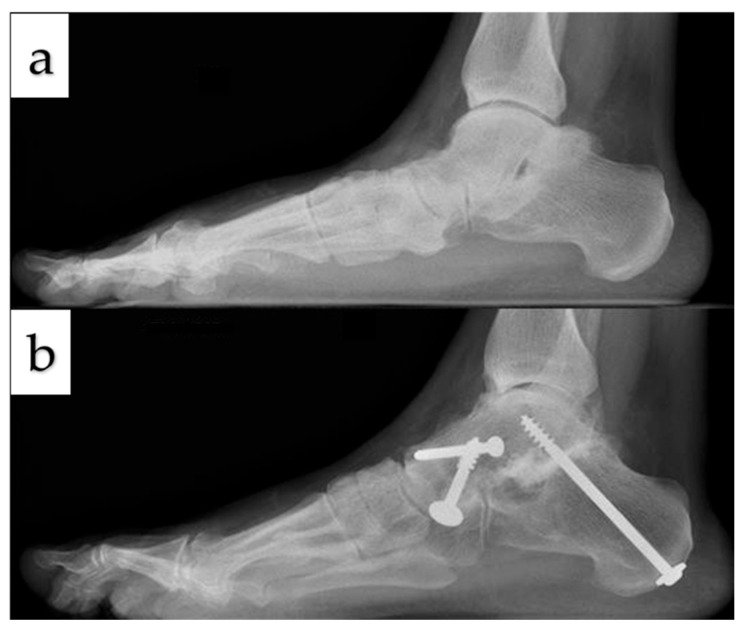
Preoperative radiographs (a) and radiographs obtained at final follow-up >10 years (b).
